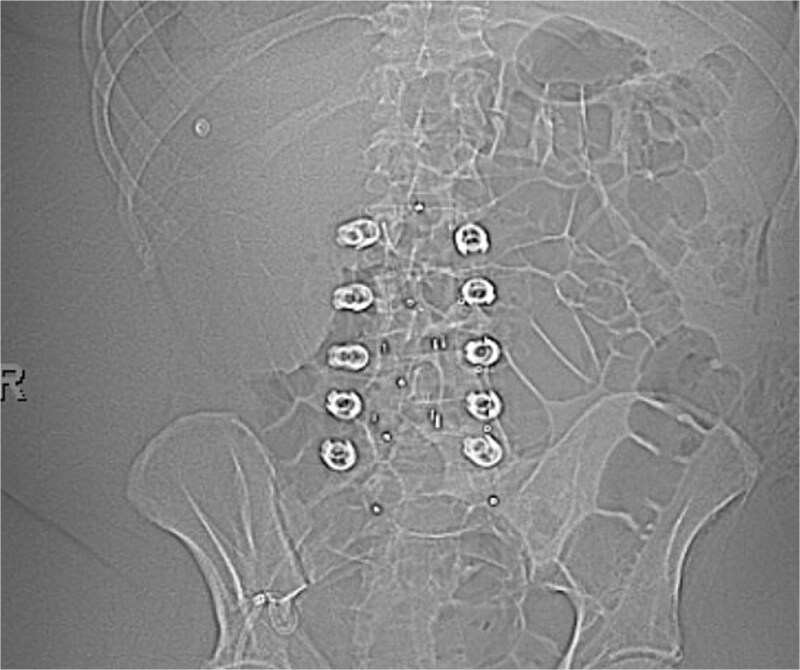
Postoperative A-P X-ray showing carbon fiber plate and screws. (AP view).
